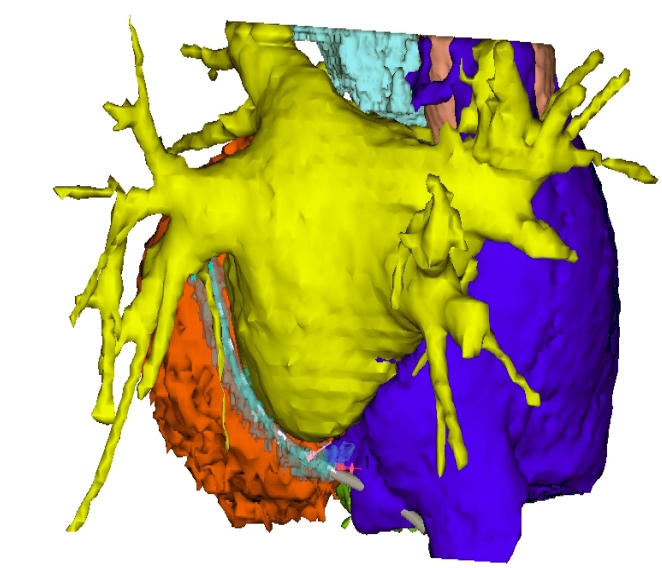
Cardiac CT derived endocardial shell of the heart reconstructed by Carto in postero-anterior view. Note the mapping catheter in the coronary sinus.
